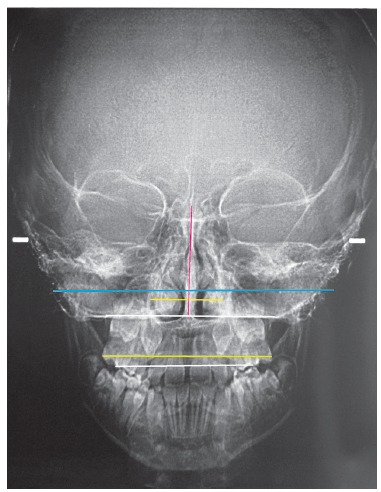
PA cephalometric radiograph post-RME, showing lines used for measurements.
